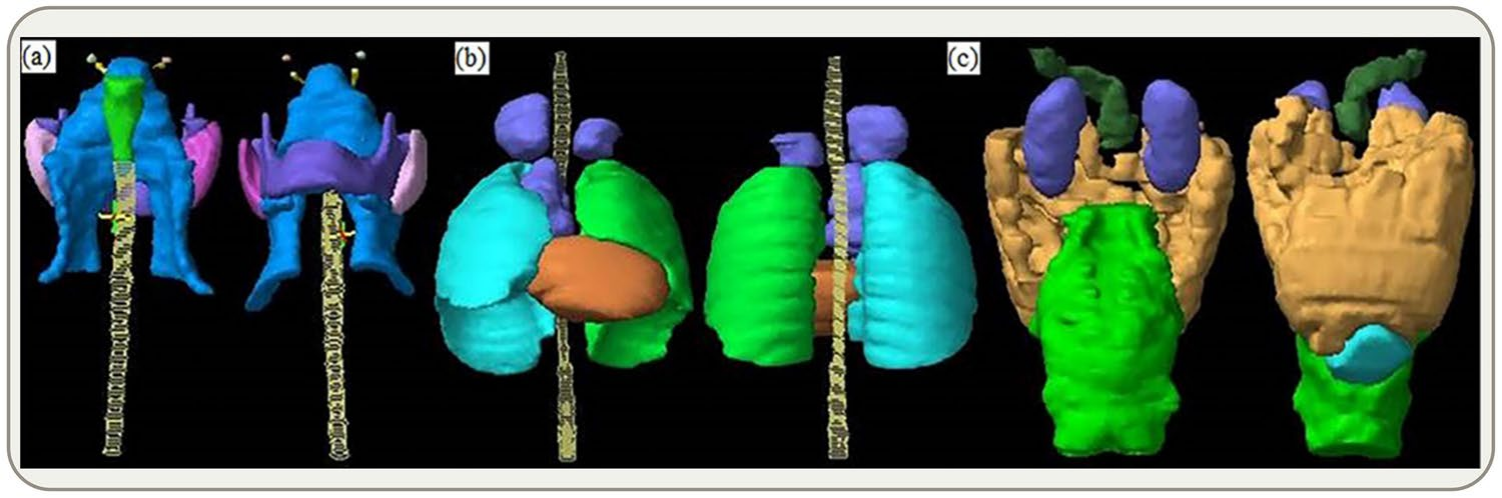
Radiation oncologists delineate PTVs and OARs on CT images. (a) PTVs are in blue, while the remaining colors indicate OARs; (b) PTVs are in light coffee, while the remaining colors indicate OARs; (c) PTVs are in light brown, while the remaining colors indicate OARs.
